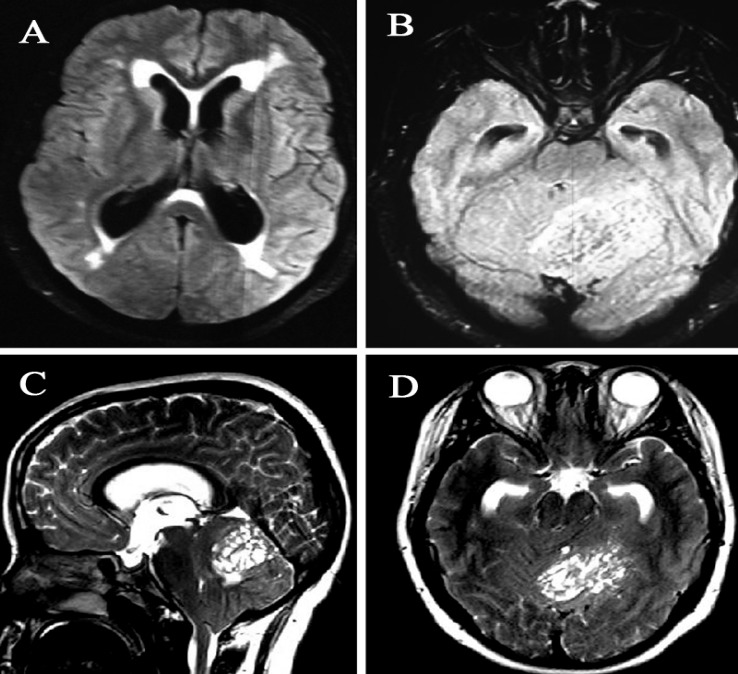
Axial T2-fluid-attenuated inversion recovery magnetic resonance imaging showing hypersignal mass in the left cerebellar hemisphere (A) and hydrocephalus due to compression of forth ventricle (B). T2-sequence MRI sagittal and axial sections showing the characteristic “tiger stripe” appearance of this hamartomatous tumor in the left cerebellar hemisphere (C and D)
